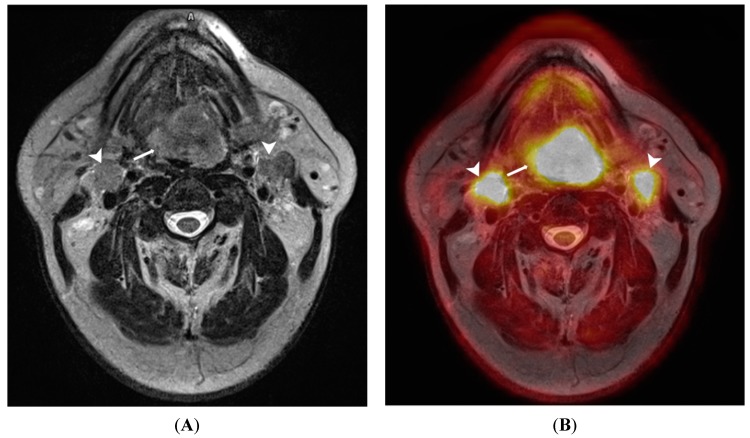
A 65-year old male patient with lingual carcinoma (A) T2-weighted MRI and (B) ^18^F-FDG PET/MRI. The primary tumour (arrows) and nodal metastases (arrowhead) are considerably more conspicuous on PET/MRI compared with T2-weighted images. This advantage could be exploited in detecting small occult head and neck tumours.
